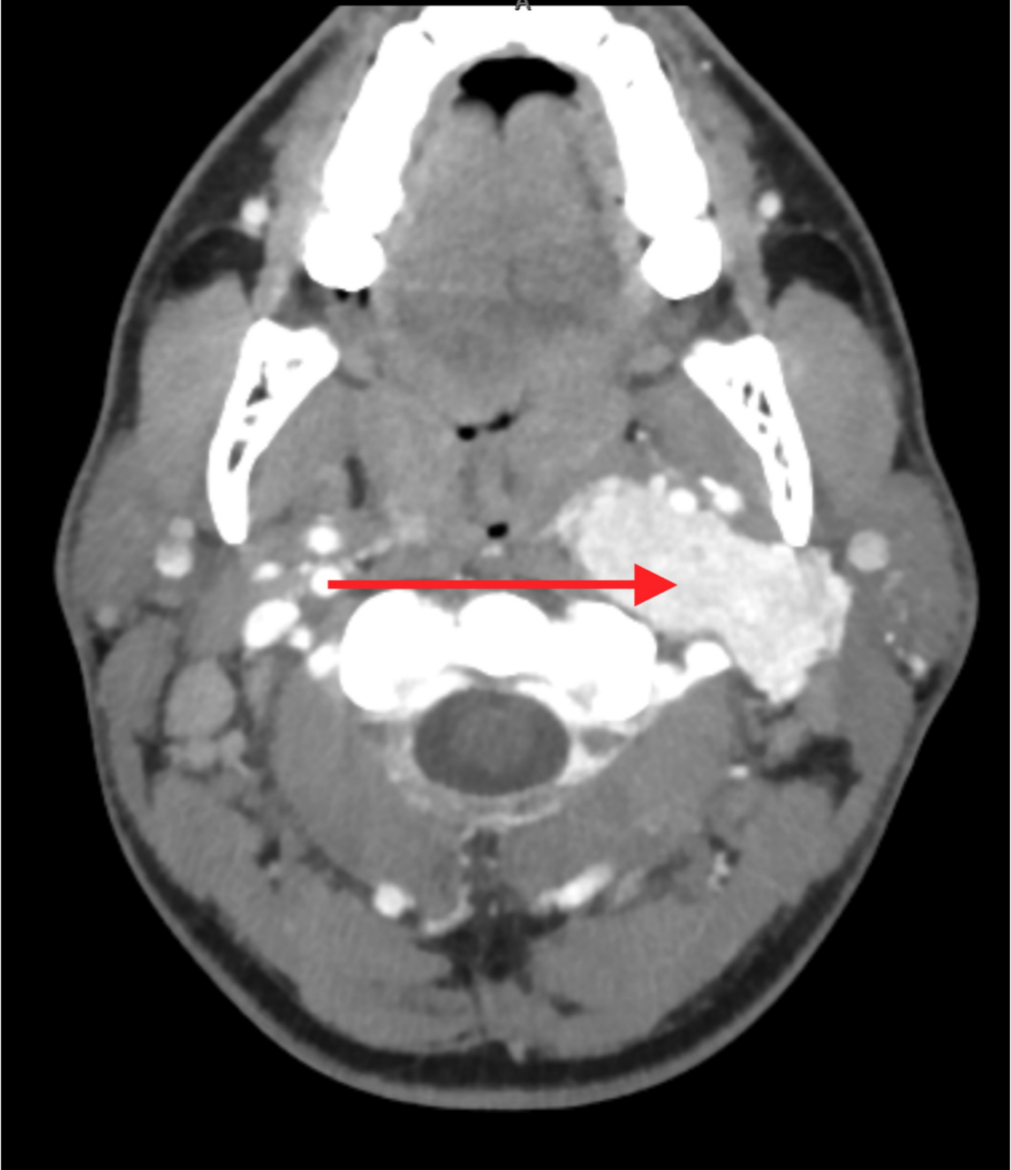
CT neck with IV contrast (axial view). Prominent pharyngeal and adenoidal tonsils with bilaterally enlarged cervical chain and submental lymph nodes. Large avidly enhancing mass within left carotid sheath.
